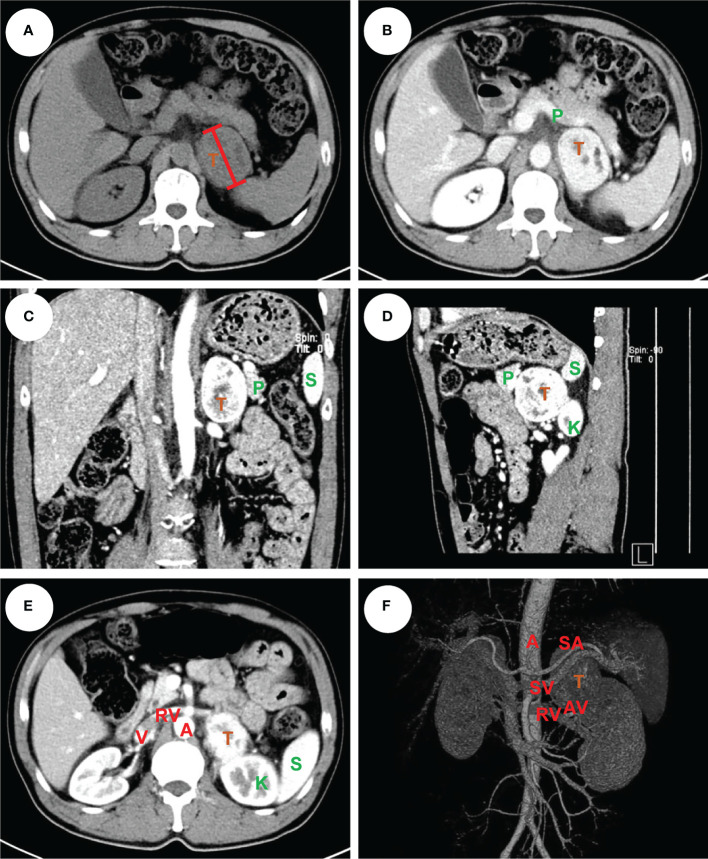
An example of left pheochromocytoma. The male patient had a 6 cm pheochromocytoma arising from the left adrenal gland (A) with 110 HU on contrast-enhanced CT (B). A clear tumor boundary was observed on transverse (B), coronal (C) and sagittal (D) section which revealed a close relationship between the tumor and ipsilateral kidney, spleen and pancreas. A venous phase section (E) showed the left renal vein travelled to the vena cava underneath the tumor, which was further confirmed by a 3-D vessel reconstruction based on arterial-venous phase CT scan demonstrating the relative location of the tumor to surrounding major abdominal vessels (F). The tumor was removed robotically *via* retroperitoneal approach. The patient had an ADRENAL score of 2_A_ + 1_D_ + 2_R_ + 2_E_ + 2_N_ + 0_A_ + L_L_ = 9L. T: tumor, P: pancreas, K: kidney, S: spleen, A: aorta, V: (inferior) vena cava, RV: renal vein, SA: splenic artery, SV: splenic vein, AV: adrenal vein.
